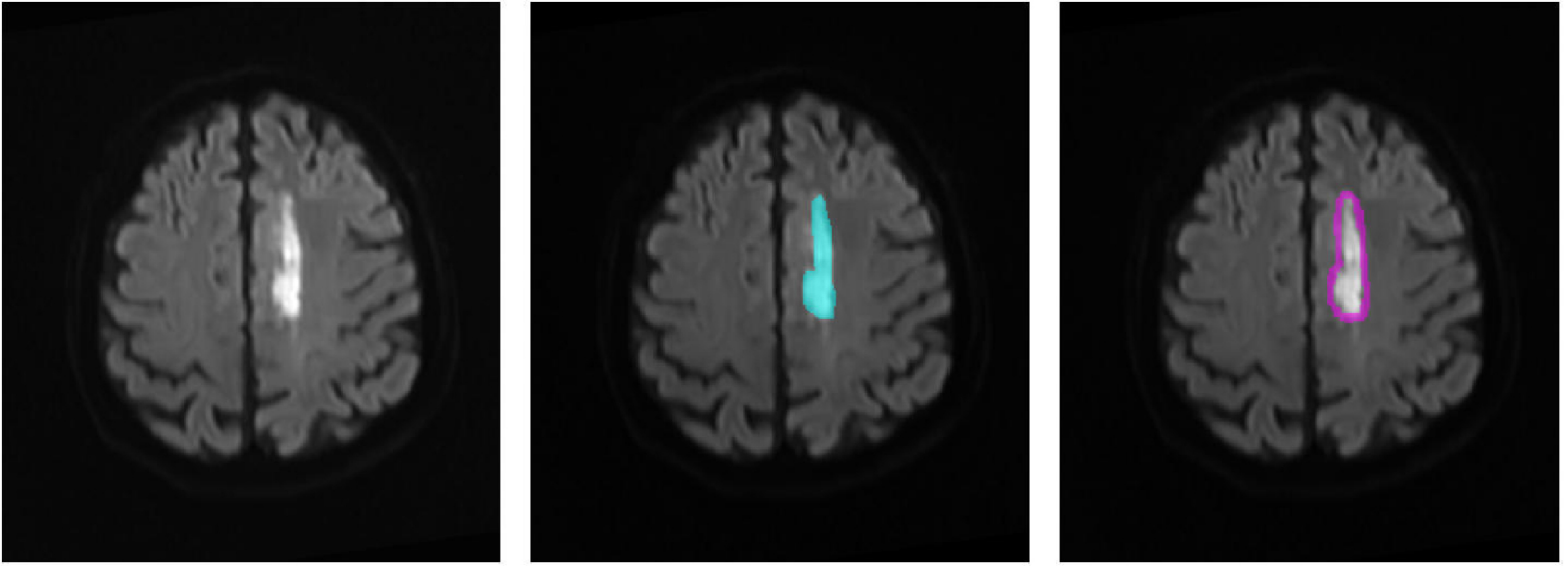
Region of interest on the DWI image. (1) The original DWI image (left), (2) the annotated ischemic lesion (middle), and (3) the delineated peri-ischemic region (right) used for radiomics analysis.
